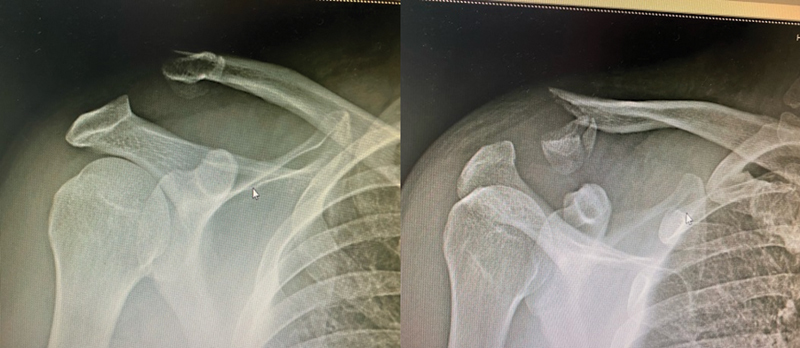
Radiograph showing the fracture of the lateral end of the clavicle with dislocation of this fragment away from the acromion and increased coracoclavicular space.
